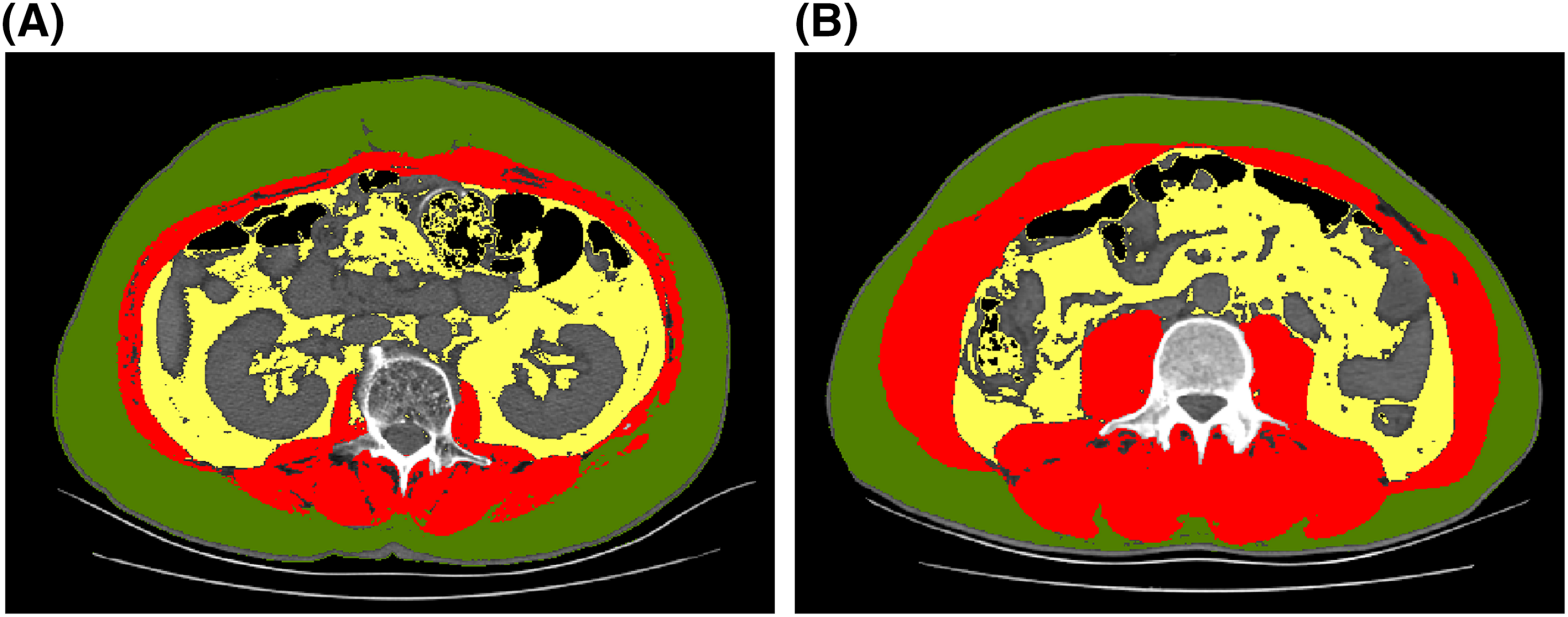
Computed tomographic scans showing areas of skeletal muscle (red), visceral adipose tissue (yellow), and subcutaneous adipose tissue (green) in patients with (A) and without sarcopenia (B).
